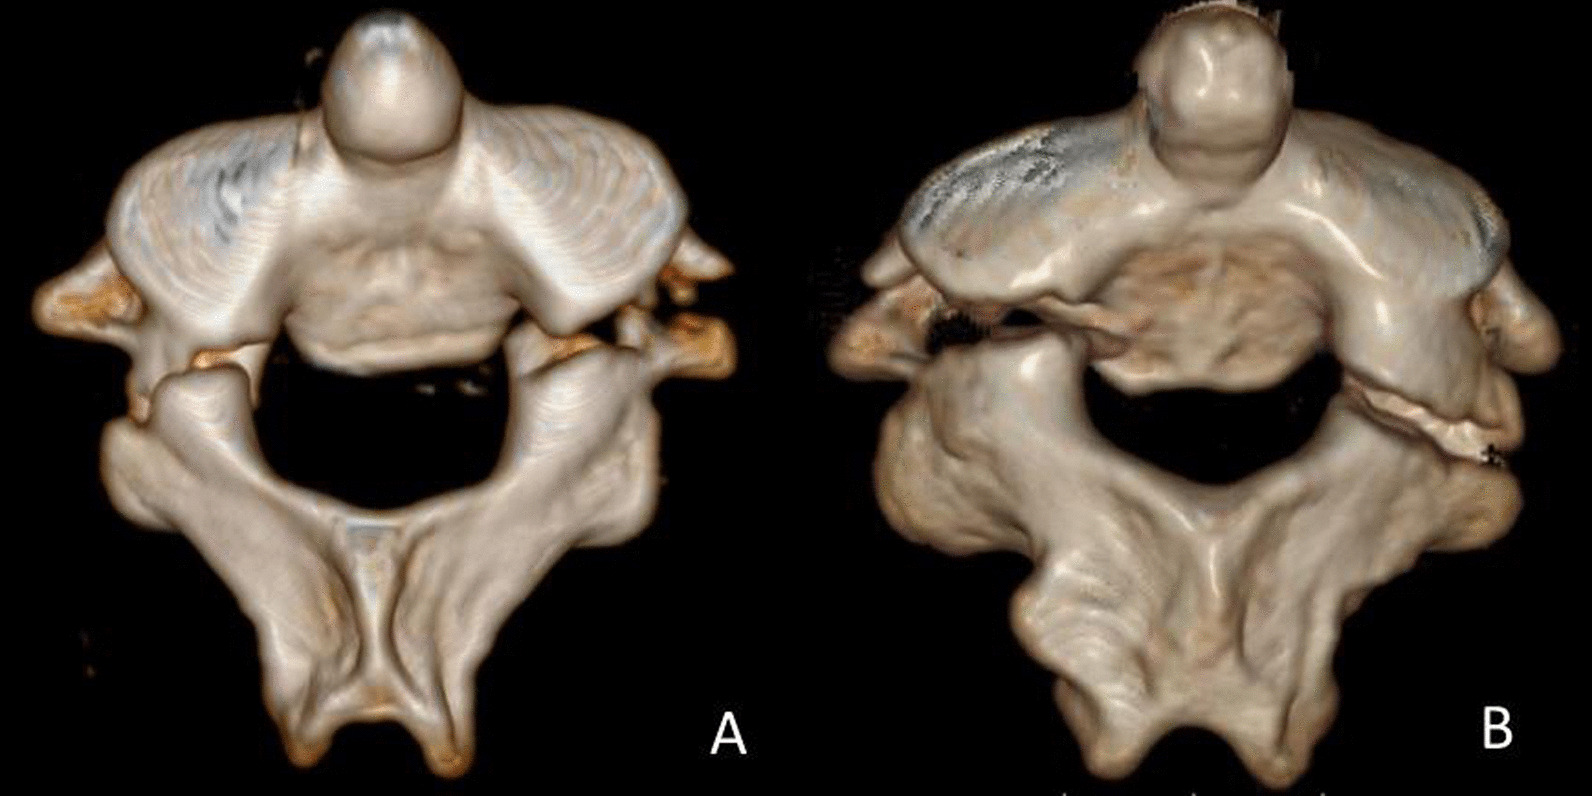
Three-dimensional reconstruction CT image showed injury of middle element on one side and another located in the middle or posterior element on the other side: A two middle elements of C2 ring, with bilateral fracture lines located on PIC; B middle and posterior elements injuries with left PIC fracture and right inferior articular process fracture
